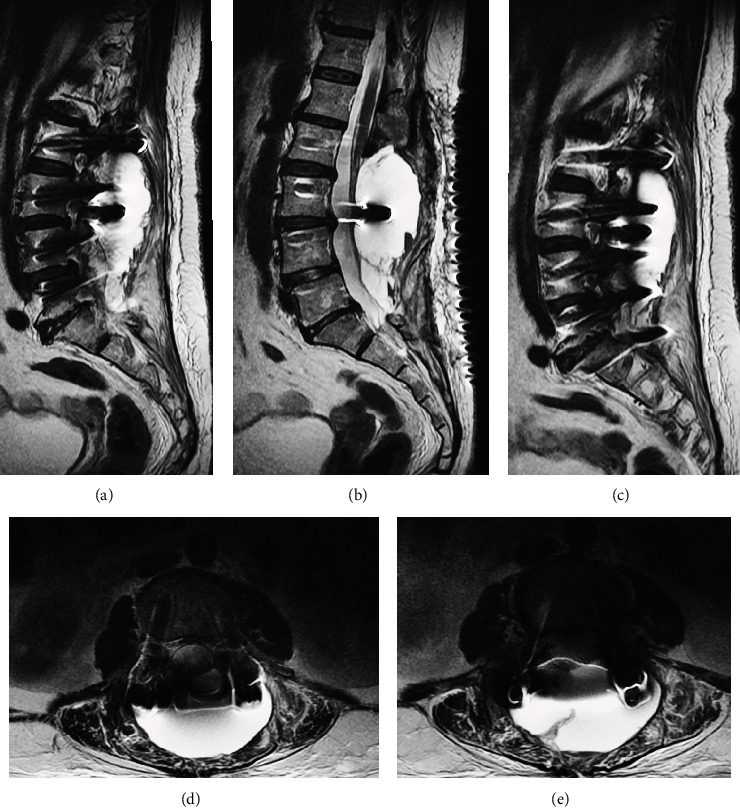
Lumbar spine MRI shows artifacts produced by metal hardware in a patient with a large pseudomeningocele. (a–c) Sagittal view. (d, e) Axial view.
